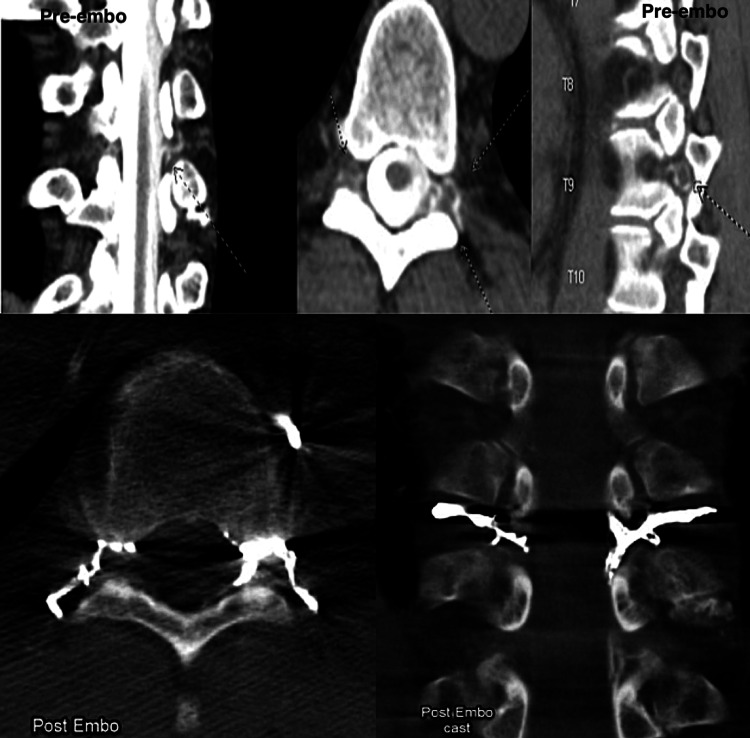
CT myelogram showing CVF suggestive of the source of CSF leak at T9-T10 CT: computed tomography; CVF: cerebrospinal fluid venous fistula; CSF: cerebrospinal fluid Top: Pre-embolization cast axial and sagittal images from CT myelography performed with the patient in lateral decubitus position showing drainage into the segmental spinal vein at T9, T10, and T11. Bottom: Post-embolization cast axial and sagittal images of CT myelography performed with the patient in lateral decubitus position showing venous embolization of T8, T10, and T11 spinal radicular veins
